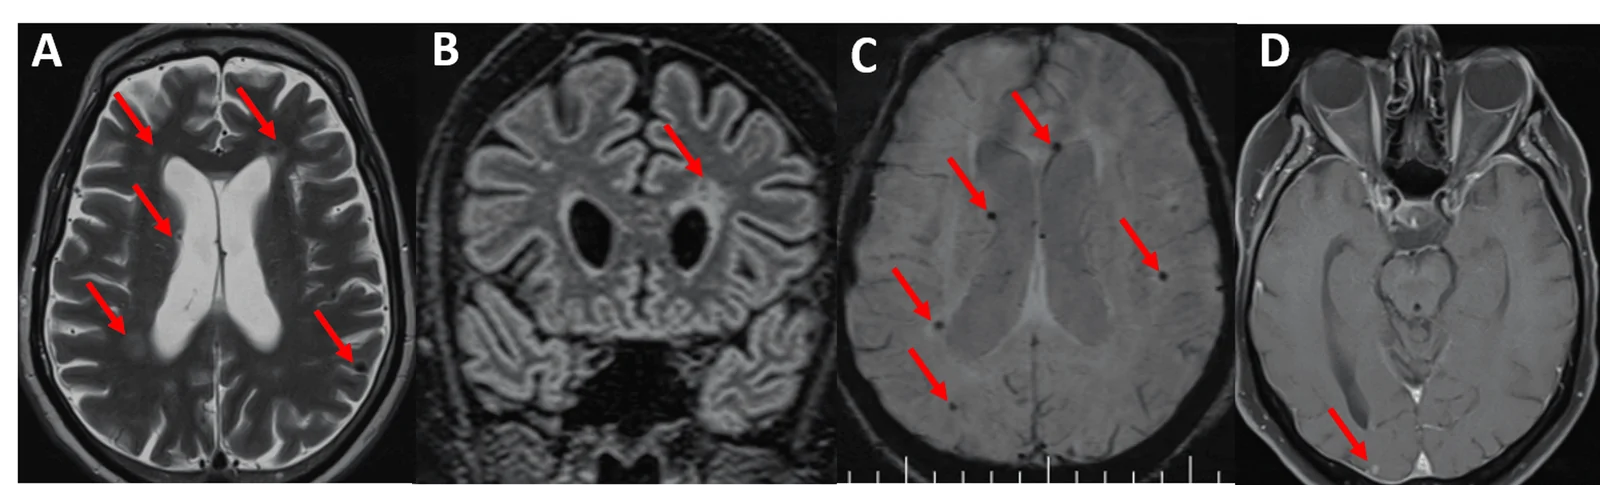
Brain magnetic resonance imaging (A) T2-weighted imaging shows rounded hypointense cortico-subcortical lesions surrounded by edema. (B) Coronal fluid-attenuated inversion recovery imaging demonstrates a periventricular lesion with a dot sign corresponding to the parasite scolex. (C) Multiple cortico-subcortical lesions demonstrate signal void on susceptibility-weighted imaging. (D) After contrast administration, enhancement of an occipital parasagittal lesion is seen.
